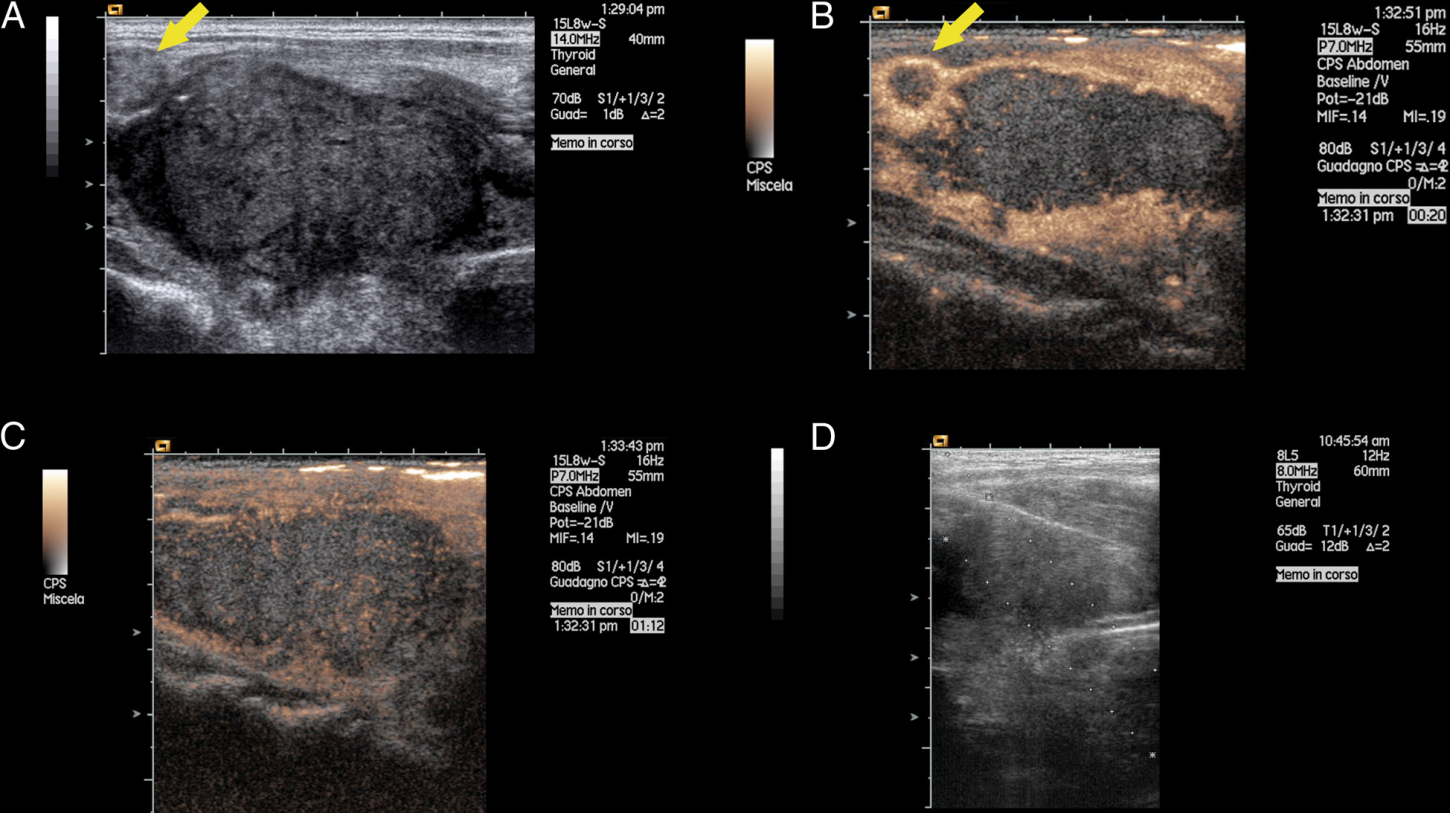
Thyroid ultrasound with contrast enhancement and biopsy. Longitudinal view of the right lobe of the thyroid gland performed with linear probe (7.5-14 Mhz) in conventional ultrasound (panel A, D) and after contrast enhancement ultrasound (panel B,C). Panel A shows a hypoechoic lesion of the right lobe of the thyroid gland. The yellow arrow indicates a metastatic lymph node located close to the upper pole of the right lobe. Panel B shows the hypovascular aspect of the nodule and lymph node after contrast injection. The enhancement is located only in the peripheral part both of nodule and lymph node as well. Panel C shows the enhancement in the venous phase which is still located only in the peripheral part of the nodule, except for the presence of small tiny vessels located also in the central part. Panel D show the path of the needle inserted inside of the nodule.
